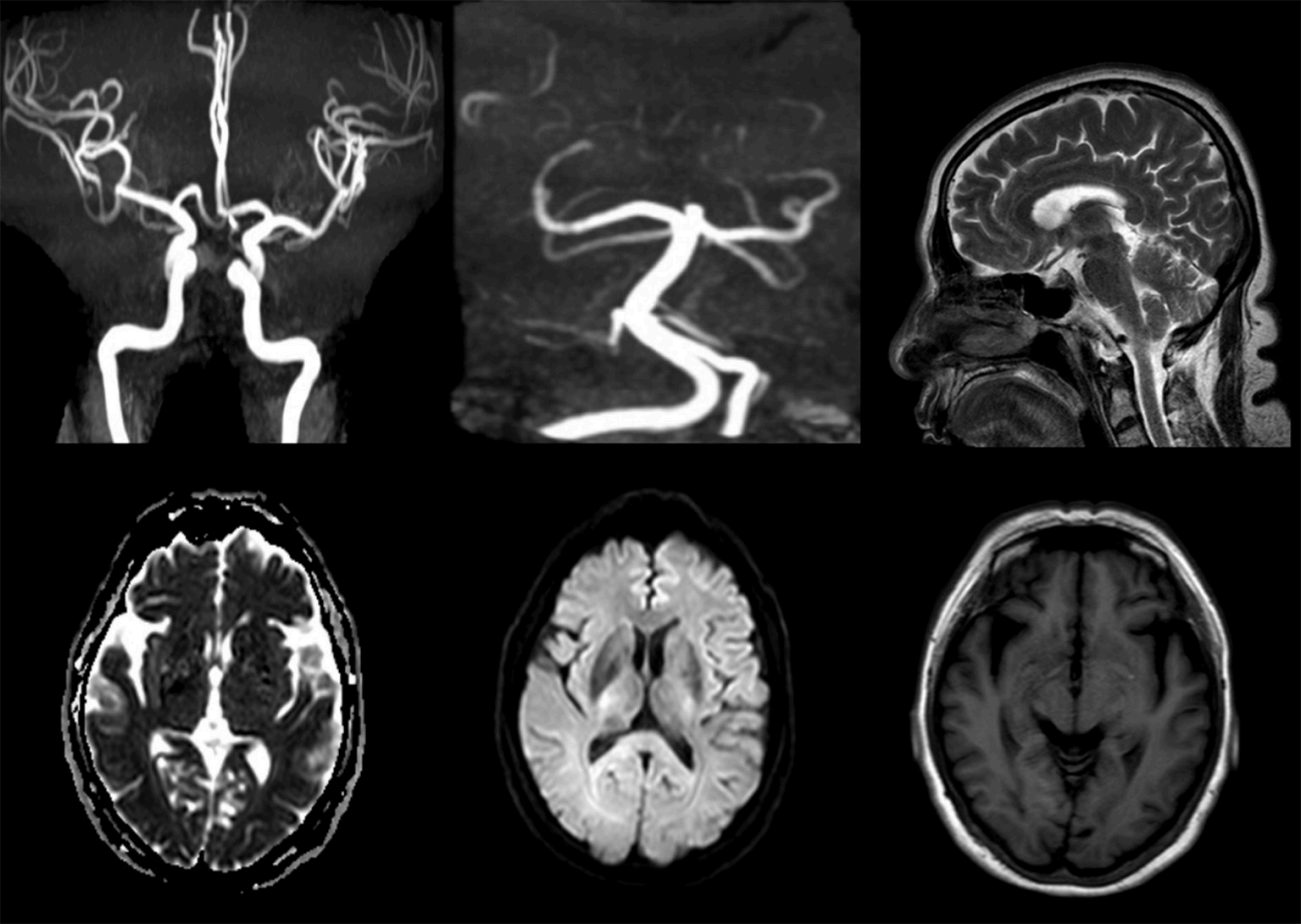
MRA and MRI images of the female patient show no meaningful abnormalities.
